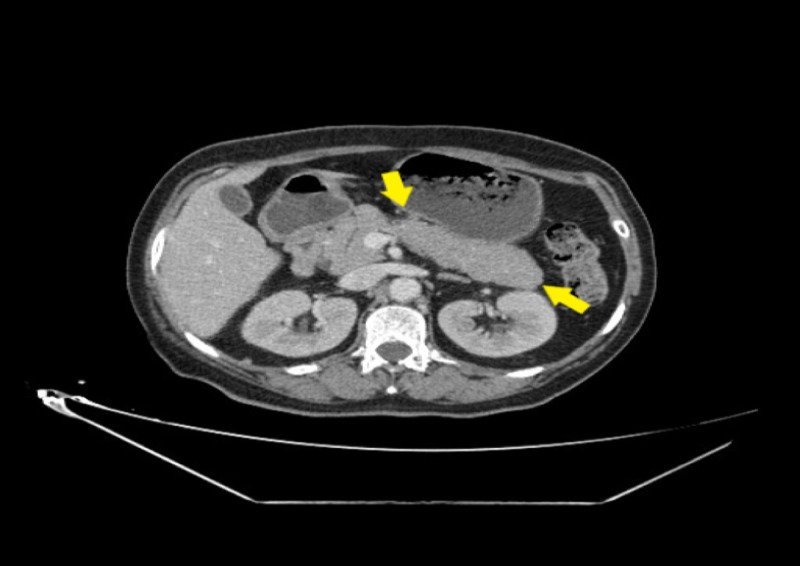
Abdominal computed tomography showing Grade B acute pancreatitis: body and tail of pancreas
